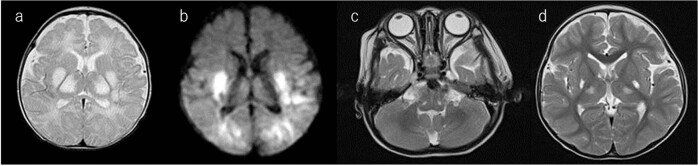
The patient’s magnetic resonance images. a, b Acute-phase brain MRI showed high-intensity signals in the bilateral thalamus, putamen and globus pallidus. c, d Follow-up brain MRI showed high-intensity signals in the bilateral thalamus, liquefaction of the dorsal putamen, and atrophy in the cortex, subcortical white matter, and white matter as sequelae of neonatal encephalopathy.
